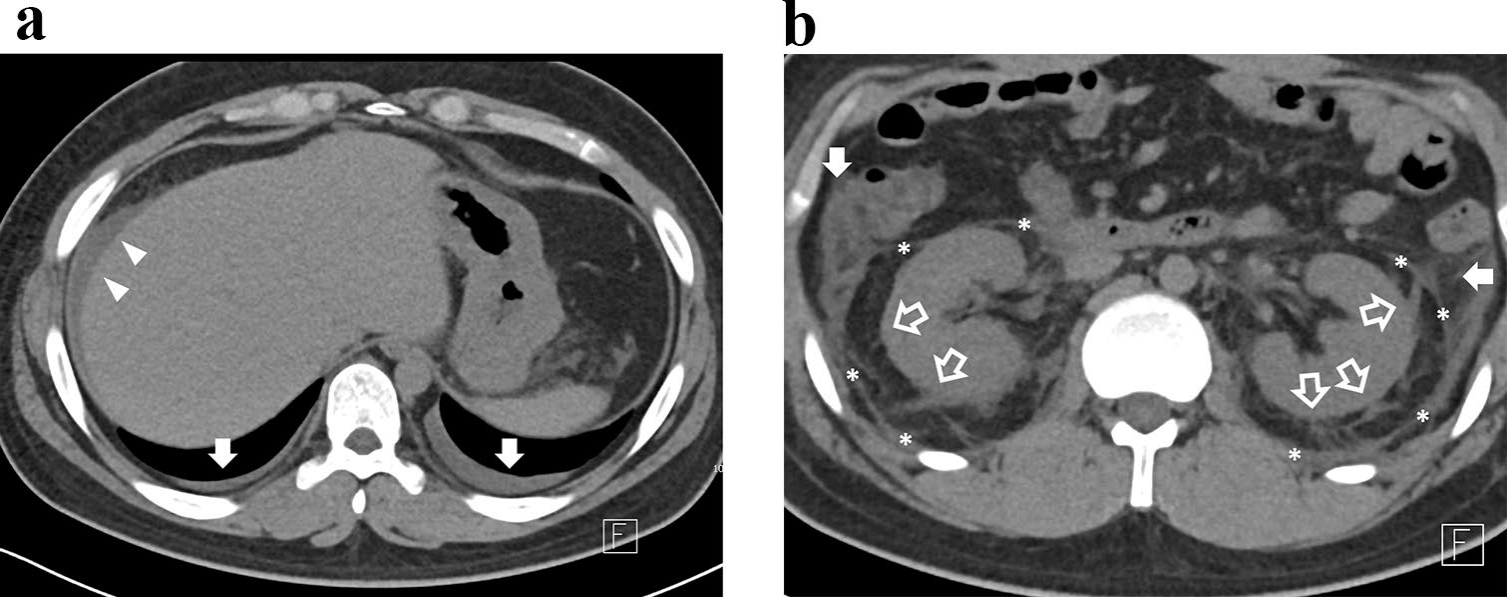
Representative CT image of a 20-year-old male patient with hemorrhagic fever with renal syndrome. A. At the diaphragm level, ascites (white arrowheads) and bilateral pleural effusion (white arrows) are noted. B. At the kidney level, perirenal fat strandings (empty arrows), pararenal fascia thickenings (asterisks) and anterior pararenal fat strandings (white arrows) are noted. The CT severity score was 5. Patient had nephrotic range proteinuria (urine protein-to-creatinine ratio of 18.7 g/g) at the time of CT examination. Hemodialysis was initiated three days following the CT scan
